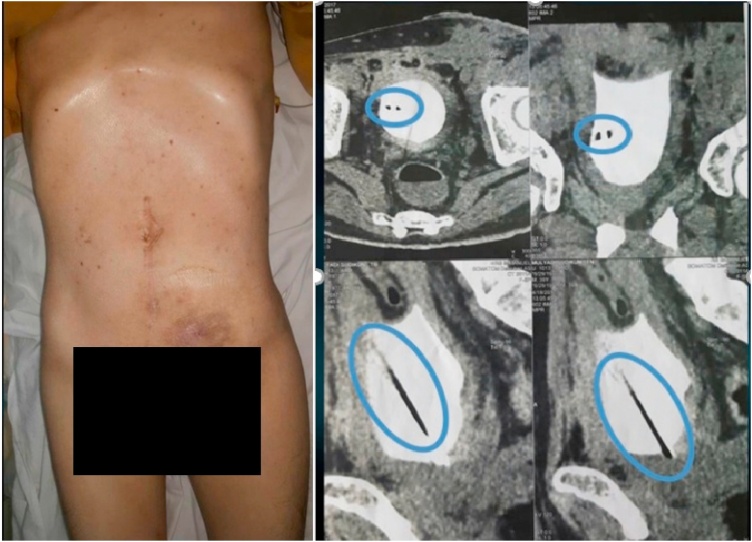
Physical examination revealed bruising on the left lower abdomen and CT-scan showed a solitary sharp-ended foreign body with one end lodged onto the bladder wall.
